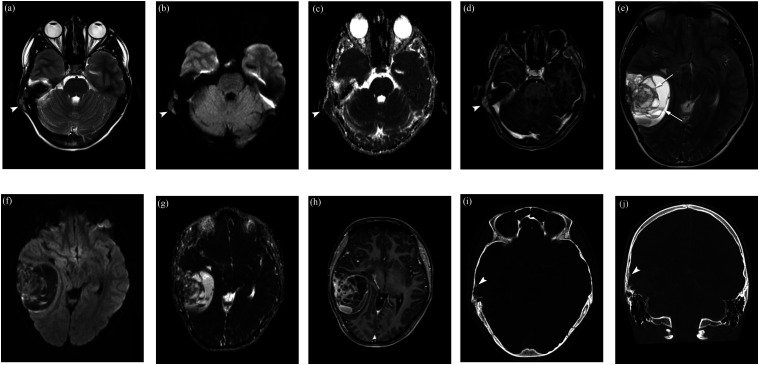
Multiparametric magnetic resonance imaging (MRI) shows a tumor arising from the squamous part of right temporal bone with a small extracranial soft-tissue mass and a large intracranial soft-tissue mass compressing the brain structures. The small extracranial soft-tissue mass (white arrowhead) reveals homogeneous intermediate signal intensity on axial T2W imaging (a), high signal intensity on axial diffusion-weighted imaging (DWI, b = 1000 s/mm^2^) with a corresponding low apparent diffusion coefficient value (ADC: 0.7 × 10^−3^ mm^2^/s) (b), (c), and homogeneous contrast enhancement on postgadolinium axial T1W imaging (d). The large intracranial soft-tissue mass mainly contains blood-filled spaces of variable size with fluid–fluid levels on T2W, axial DWI (b = 1000 s/mm^2^), and ADC images (e)–(g), and smooth septal and rim contrast enhancement (h), suggesting ABC-likes changes (white arrows). Axial and coronal CT (i), (j) images show an ill-defined, permeative bone destruction in the squamous part of right temporal bone. Aggressive spiculated periosteal reaction and expanded cortex with Codman triangle (white arrowhead).
